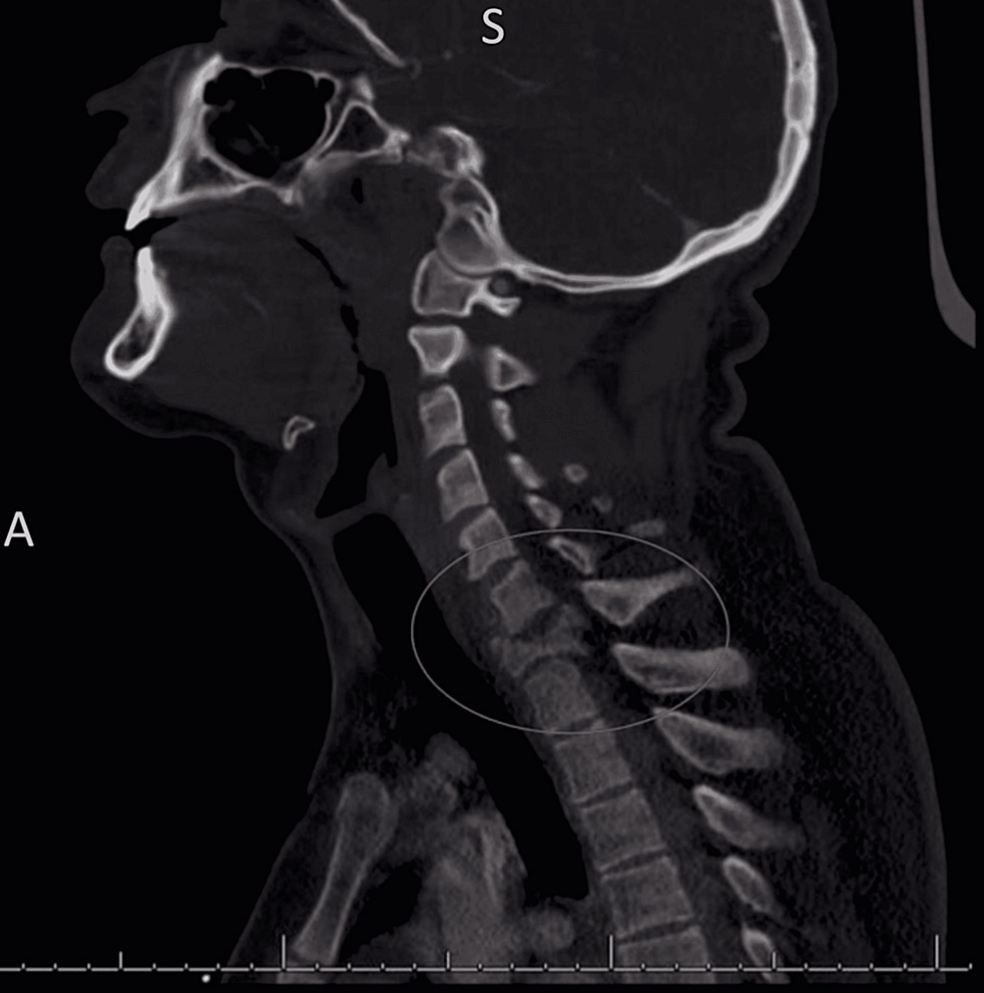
CT cervical spine without contrast showing unstable C7 burst fracture with retropulsion and severe cord compression. CT, Computed Tomography
